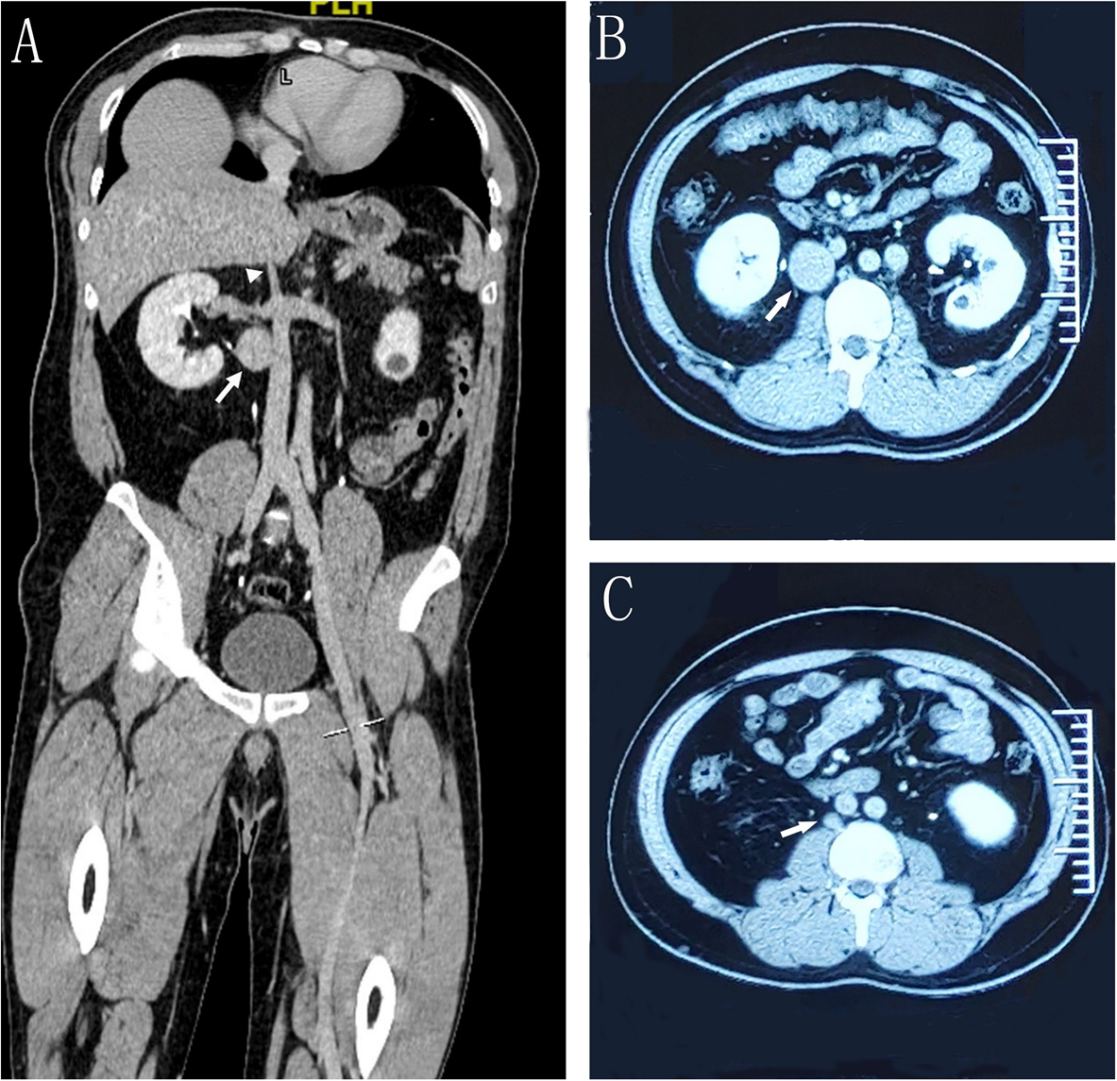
Contrast-enhanced CT imaging. a Coronal view showing the stenosis of IVC (arrowhead) and a retroperitoneal mass on the right side of the infrarenal IVC (long arrow). b and c Axial view showing the two retroperitoneal mass (long arrow)
